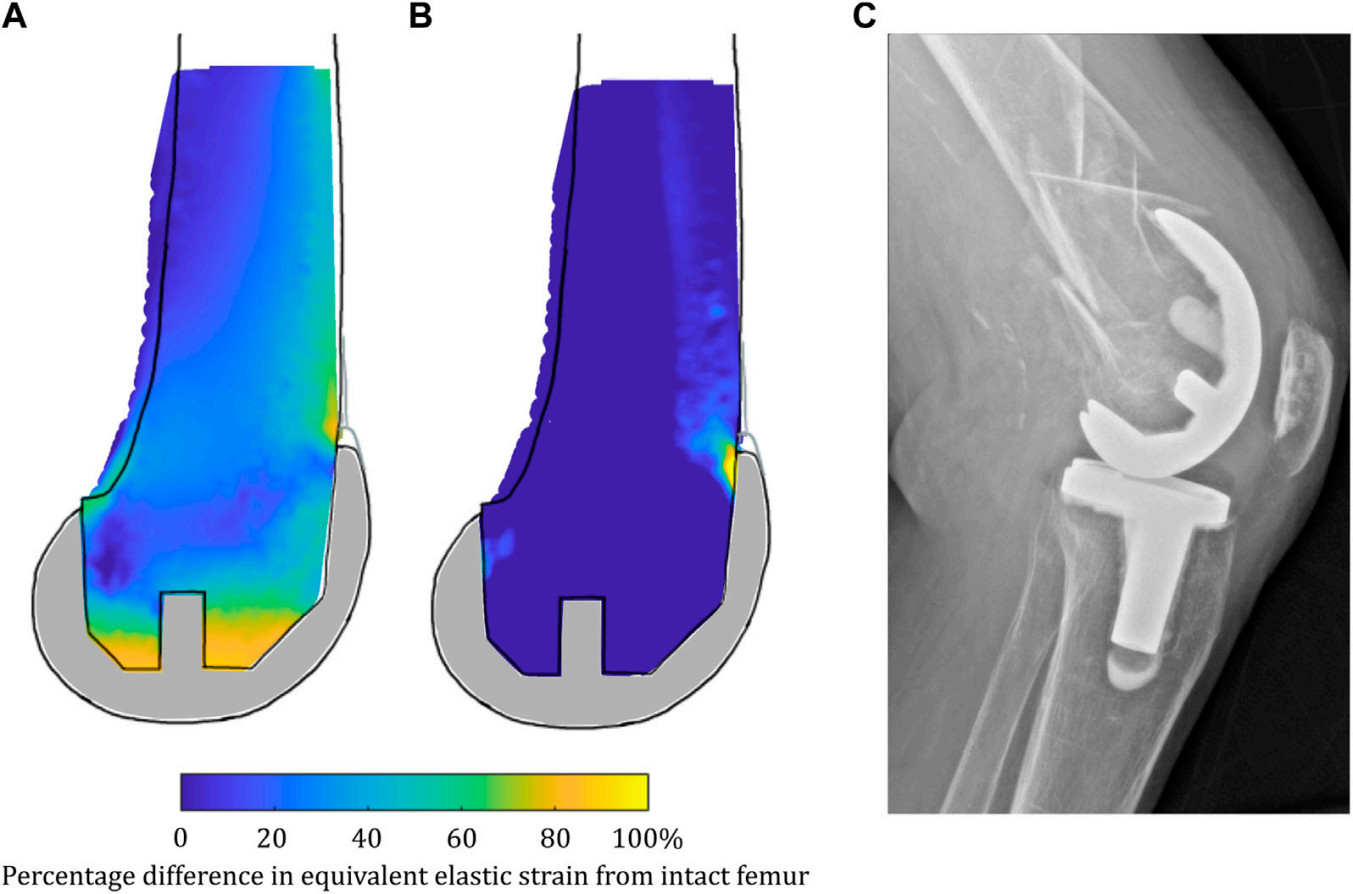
(A) Strain shielding during low-flexion gait combined with (B) overstraining during higher-flexion stair ascent in a sagittal slice through the lateral femoral condyle when implanted with TKA may contribute to periprosthetic femoral fractures such as that shown in (C) a lateral radiograph of a femoral TKA periprosthetic fracture.
